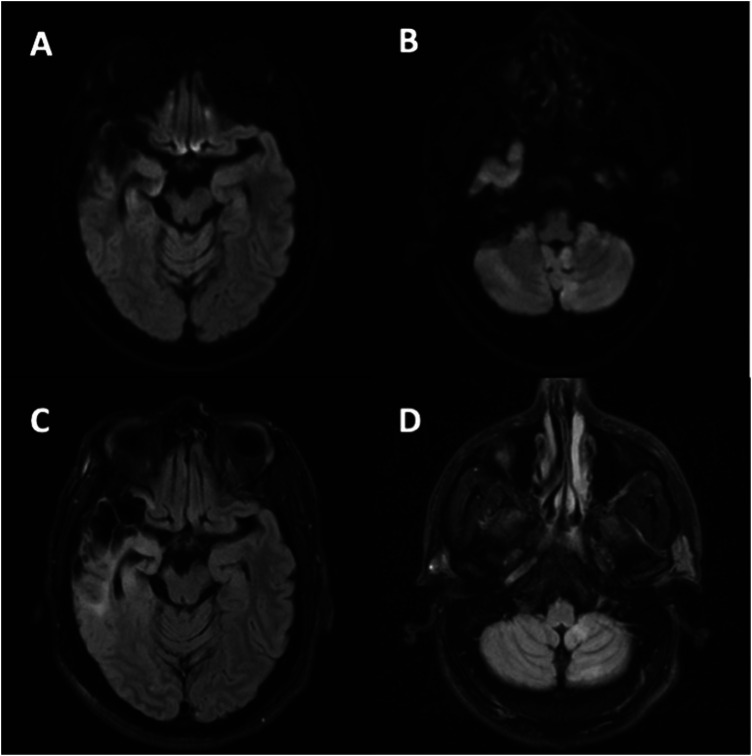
Repeat Brain MRI 3 days after seizure cessation*: Resolution of previously noted restricted diffusion (A), (B) and T2 FLAIR hyperintensity (C), (D) in the right temporoparietal cortex (A), (C) and left cerebellar hemisphere (B), (D).
